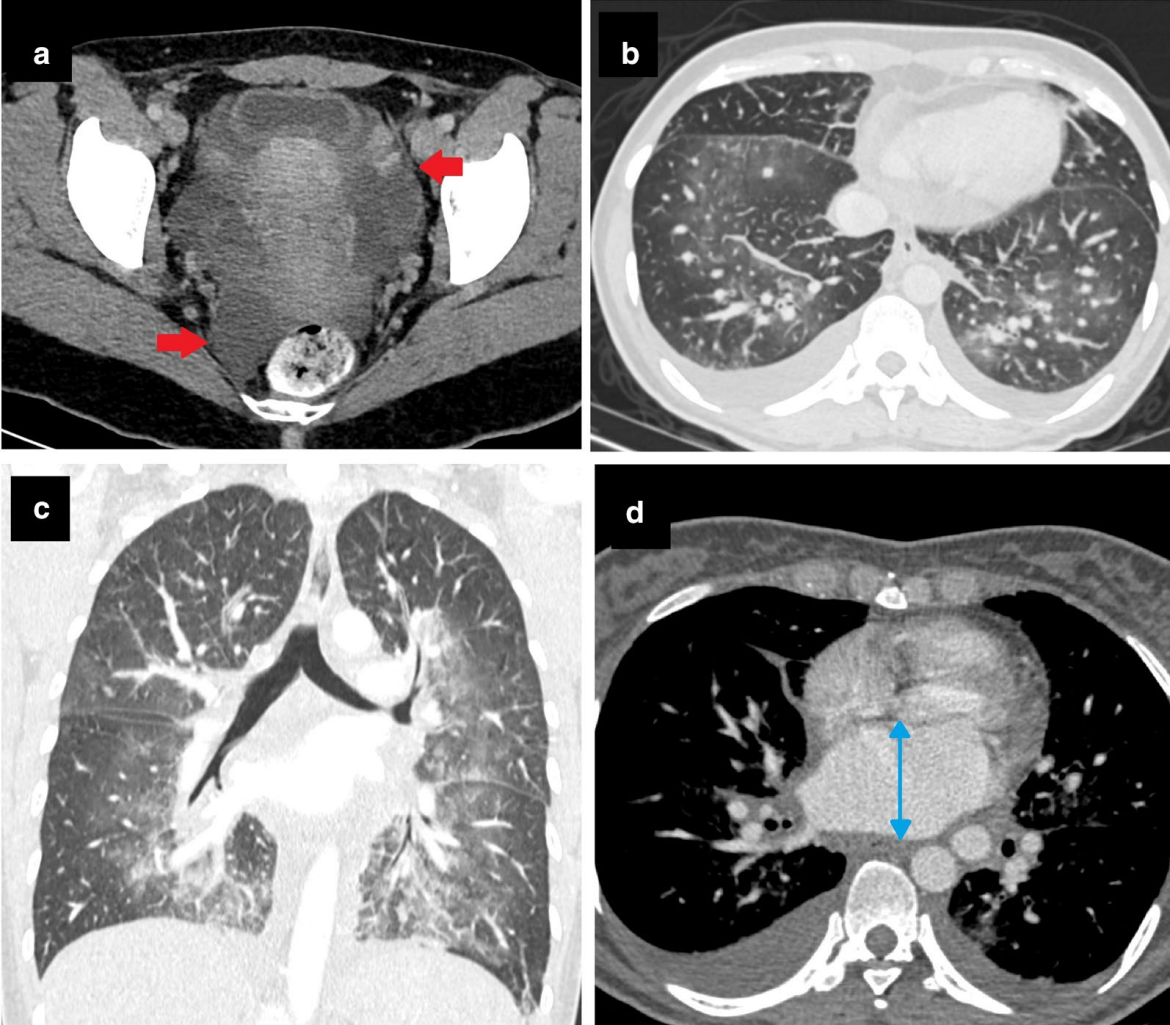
Poor communication (type 5) error with attribution bias. A 27-year-old female patient with no known disease presented at the Emergency Department with abdominal discomfort. After the first evaluation, an abdominal CECT was performed (a, b). Bilateral lung bases were also partially involved in abdominal CECT (b). CT was reported as “free fluid in the pelvis (red arrows a), bilateral pleural effusion, interlobular septal thickenings, and bilateral central ground-glass opacities in lung bases” (b). Although these are well-known findings of cardiac congestion, they were not attributed to cardiogenic edema because of the patient’s age and clinical history. Instead, findings were only described without any comment or impression in the report, and clinicians were expected to read the report and evaluate findings. Six hours later, the patient became dyspneic and tachypneic. Contrast-enhanced thorax CT (c, d) revealed findings compatible with pulmonary edema (c) and enlarged left atrium (blue double-sided arrow, d). Echocardiography, performed to rule out cardiac abnormality, showed severe mitral stenosis, possibly due to rheumatic heart disease. Attribution bias due to the “young age, clear medical history and irrelevant symptoms of the patient” caused the radiologist to avoid making any comments about the possibility of cardiac congestion in the report. As a result, poor communication between the radiologist and clinicians due to the lack of necessary interpretation in the report caused a delay in diagnosis
